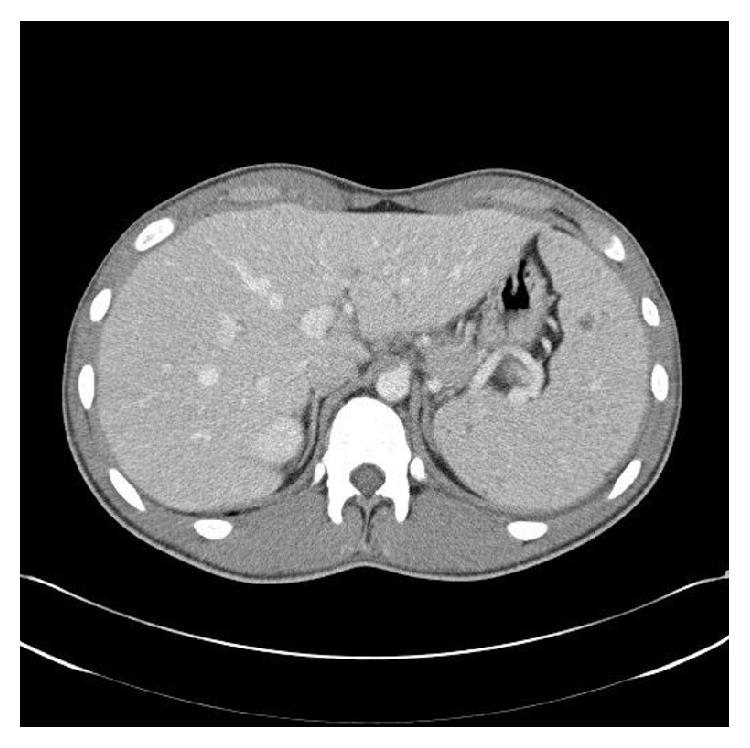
Computer tomography (CT) of the chest and abdomen. Transverse views showed multiple hepatic and splenic lesions.
